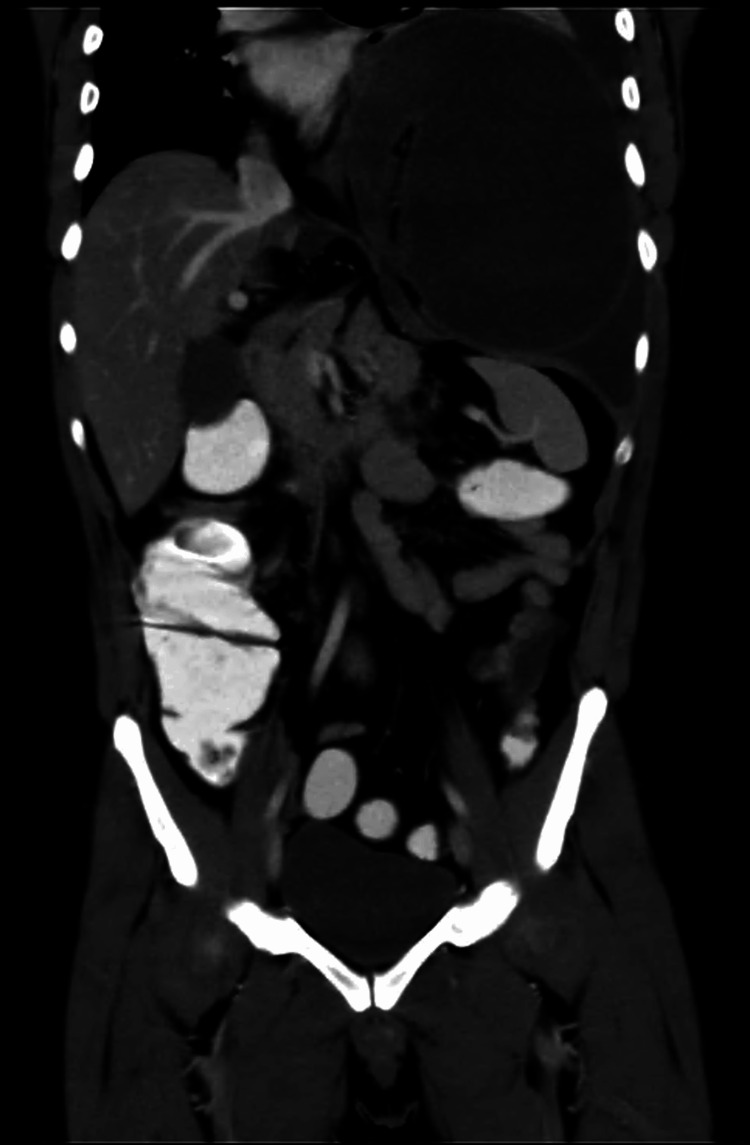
Coronal view of CT scan showing the stomach herniated into the chest.
